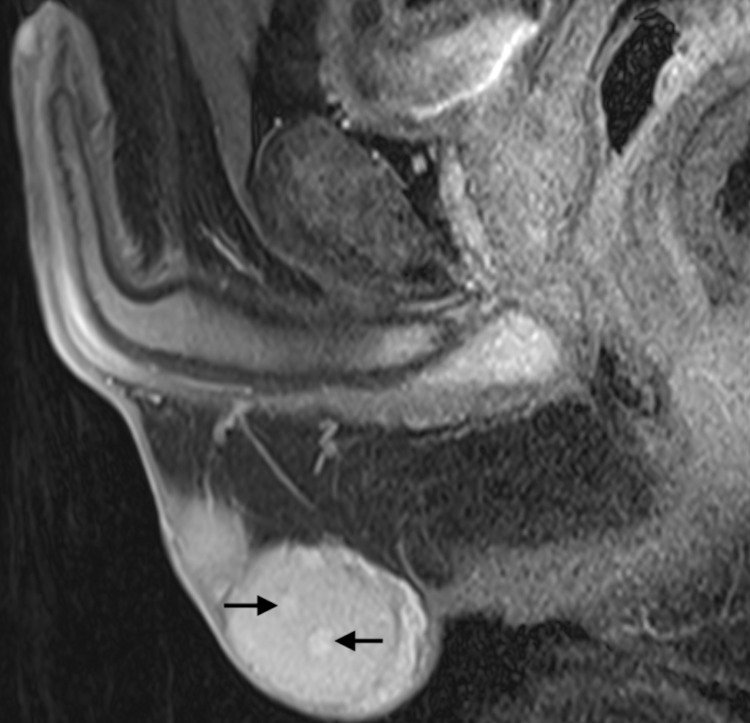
T2-weighted MRI shows multiple nodular lesions below 6 mm in size, with weak heterogeneous contrast uptake with arrows.
